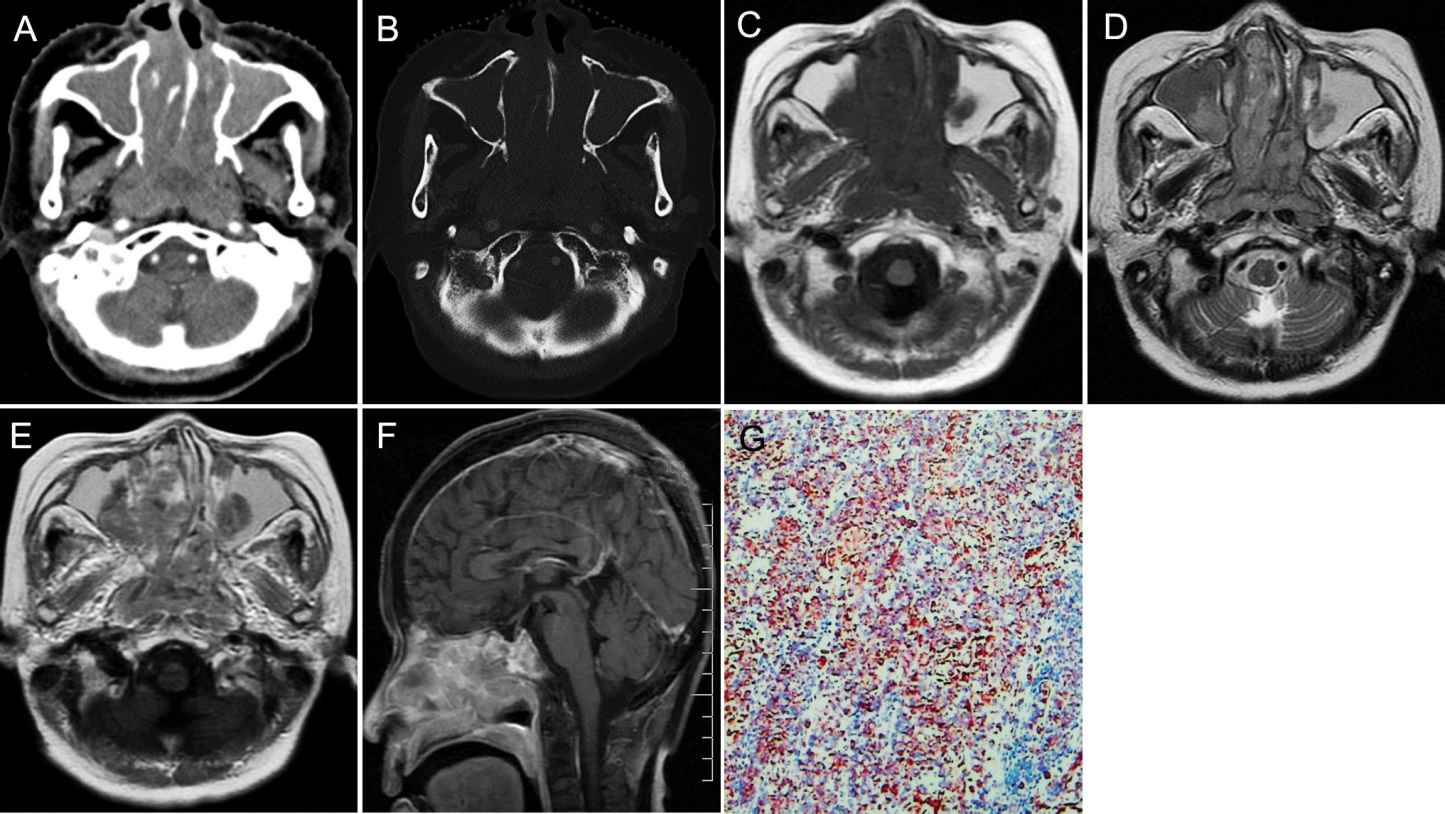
pPNET in a 63-year-old man CT imaging showed an irregular nasopharynx mass with ill-defined borders. The mass had uneven enhancement (A). The sphenoid pterygoid demonstrated bone resorption (B). Precontrast MRI showed a hypo-intense mass on T1WI (C) and hyper-intense mass on T2WI (D). Enhanced MRI showed significant and heterogeneous enhancement (E). Sagittal enhanced MRI (F) images showed the mass invaded the slopes and anterior cranial fossa. The small round tumor cells were positive for CD-99 (G × 100).
